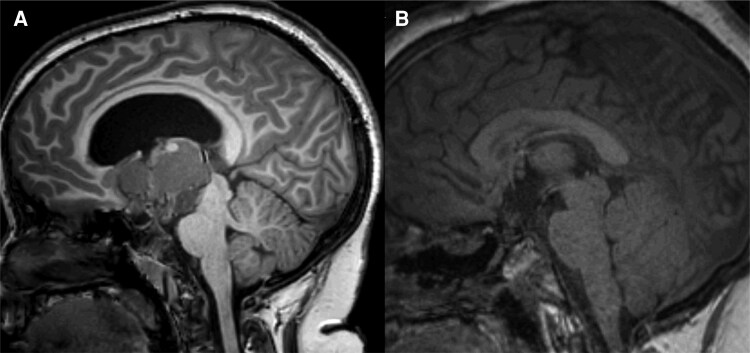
A, Case magnetic resonance imaging (MRI), pre procedure (left panel). A complex cystic and solid suprasellar mass is demonstrated, extending into and expanding the third ventricle. The mass contains central coarse calcifications, enhancing solid components, and numerous cysts of varying signal intensity. The mass measures approximately 4.7 × 4.2 × 4.6 cm (AP × TRV × CC). The optic chiasm is compressed and displaced anteriorly by the mass, with edema extending throughout the optic chiasm and into the prechiasmatic optic nerves. There is surrounding T2 hyperintensity extending into the hypothalamus and posterior limbs of the internal capsules bilaterally. The thalami and cerebral peduncles are splayed by the mass. No distant foci of nodularity or abnormal enhancement are identified. The cerebral sulci are diffusely small in size. The basal cisterns are narrowed but appear patent. There is no evidence of intracranial hemorrhage, restricted diffusion, or extra-axial fluid collection. The pituitary gland appears flattened along the floor of the sella, with nonvisualization of the posterior pituitary bright spot. B, Case MRI, post procedure (right panel). Prior transsphenoidal approach for resection of craniopharyngioma centered in the suprasellar cistern, with evolving postsurgical changes. Stable appearance of signal abnormality involving suprasellar and interpeduncular cisterns, inferior third ventricle, fornices, and anterior commissure, as described. Continued surveillance is recommended to ensure stability of these regions. No evidence of new or worsening parenchymal signal abnormality. Bifrontal encephalomalacia, particularly involving the parasagittal anterior frontal lobes. Bifrontal approach ventricular catheters, terminating in the frontal horns of the lateral ventricles, unchanged. Decompressed ventricular system, without evidence of hydrocephalus.
